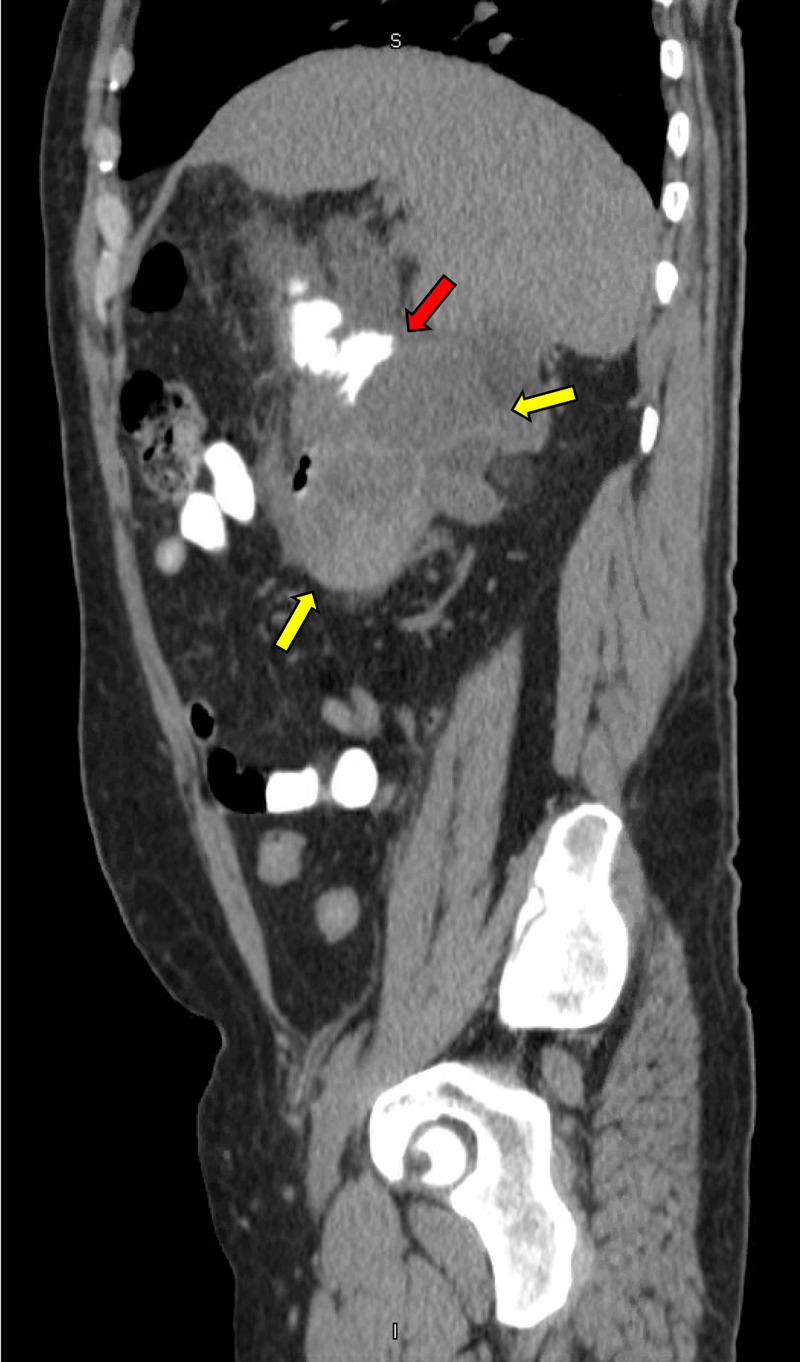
CT scan of the abdomen and pelvis sagittal plane. Right retroperitoneal lobulated masses (yellow arrows) provoking duodenal obstruction (red arrow).
